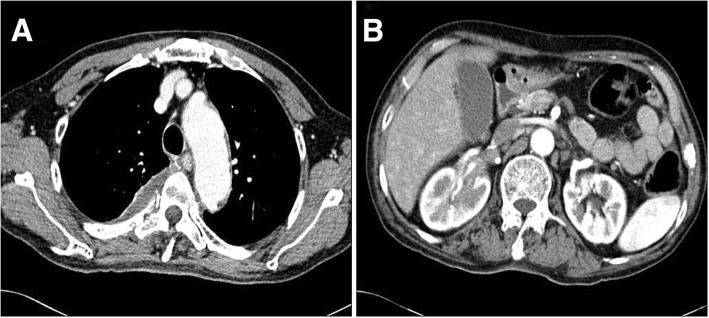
Contrast-enhanced computed tomography after 1 month of treatment. a Contrast-enhanced chest computed tomography shows reduction in size of the pleural mass. b Contrast-enhanced abdominopelvic computed tomography shows decreased infiltration around the right renal pelvis (longest diameter, 31 mm)
